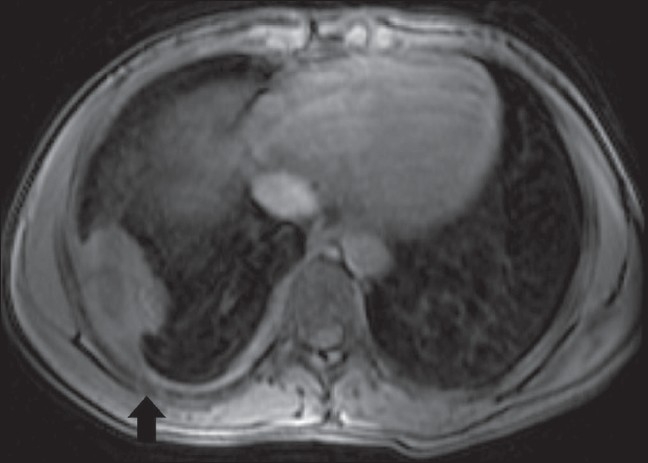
Chest wall tuberculosis. Axial, contrast-enhanced, fatsuppressed, T1W MRI shows a pleural-based mass along the dorsal aspect of the right thoracic wall, with irregular peripheral enhancement. Note the hazy contours of the adjacent rib (arrow) due to osteomyelitis
